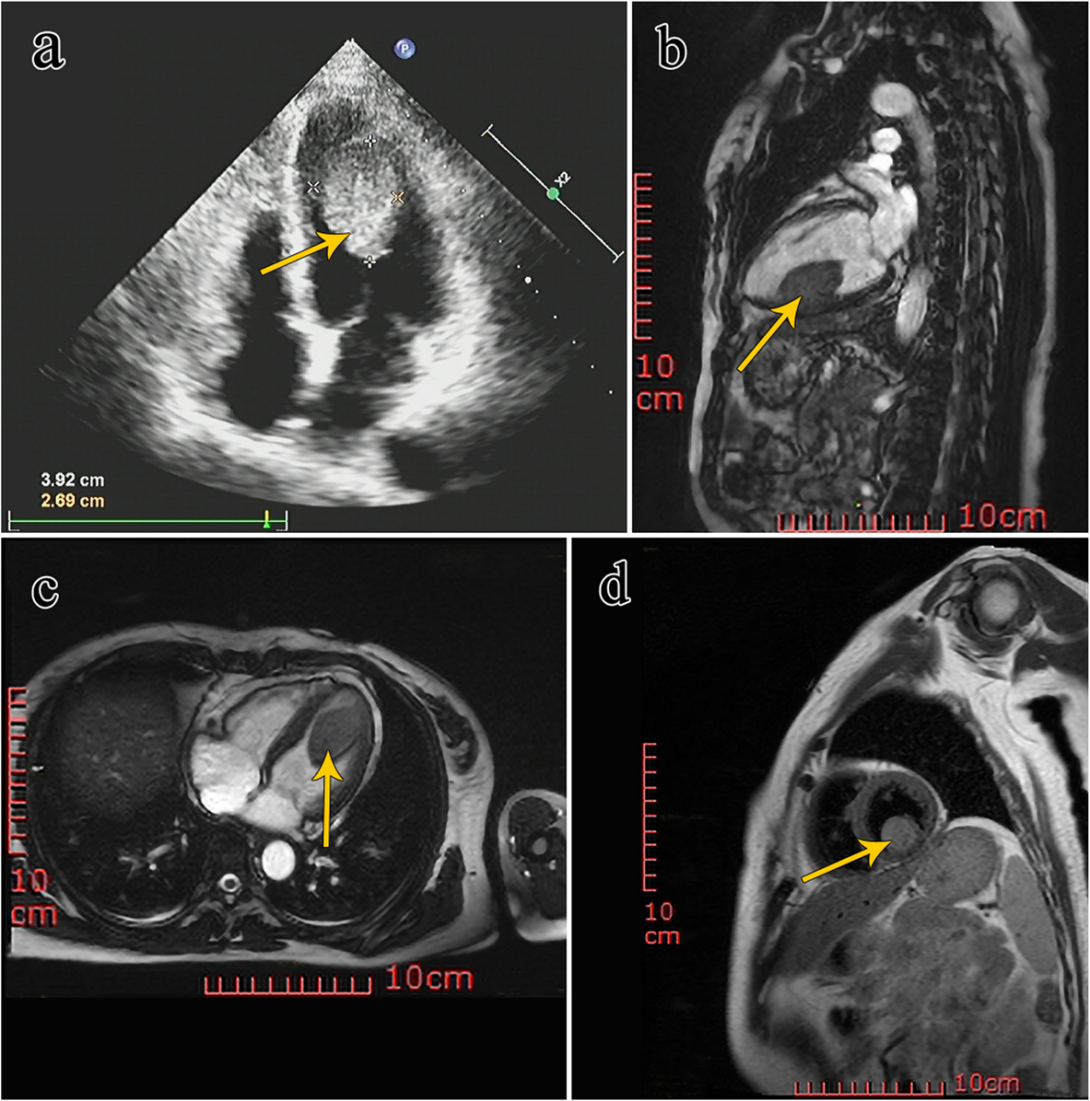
a Echocardiography showed a homogeneous mass in the left ventricle (the yellow arrow). b, c Magnetic resonance imaging (MRI) showed a longer T2 signal of the left ventricular mass (the yellow arrow). d MRI demonstrating round mass with clear boundary in left ventricle (the yellow arrow)
